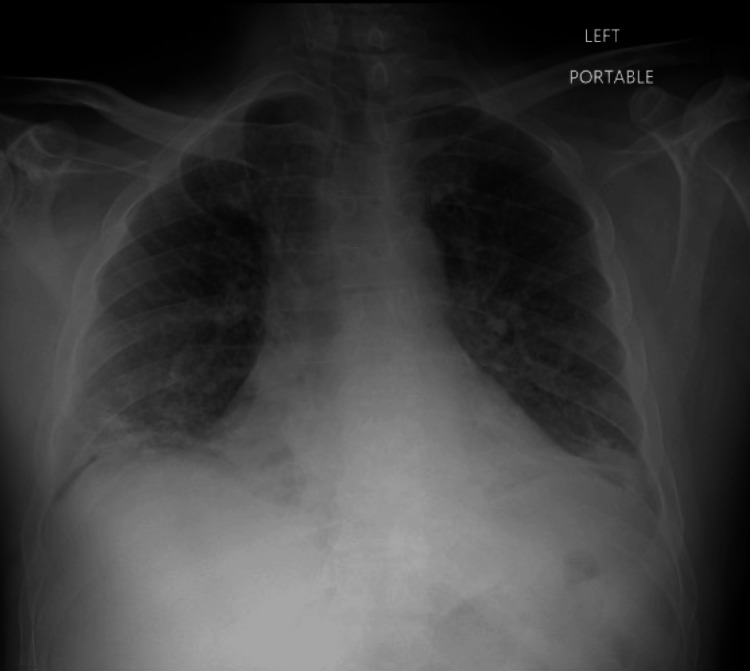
X-ray chest showing interstitial pulmonary edema
